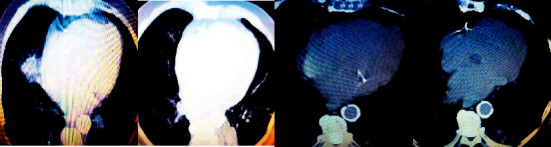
CT image data of a patient with COPD. Male, 75 years old, cough, shortness of breath, bloody expectoration, and sometimes unable to breathe and woke up at night.
